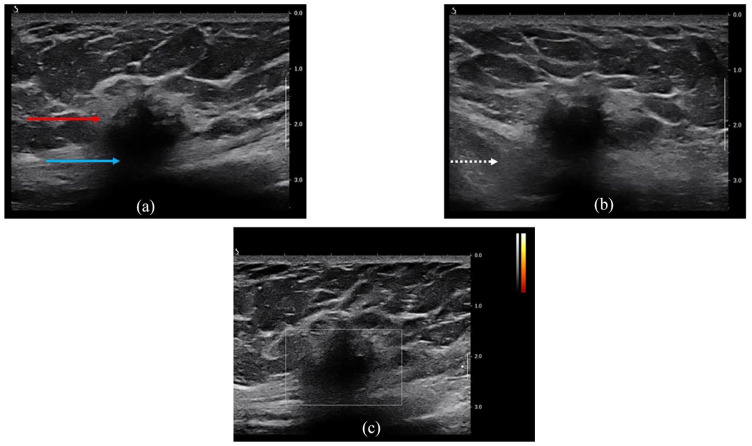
High-resolution real-time ultrasound of the right breast reveals a 1.5 × 2.1 × 1.8 cm irregular hypoechoic mass (red arrow) with spiculated margins and posterior shadowing (blue arrow) in the lower inner quadrant at 5 o’clock, located 3 cm from the nipple (a). The mass abuts the pectoralis musculature (dashed white arrow) (b). No internal vascularity was observed (c).
